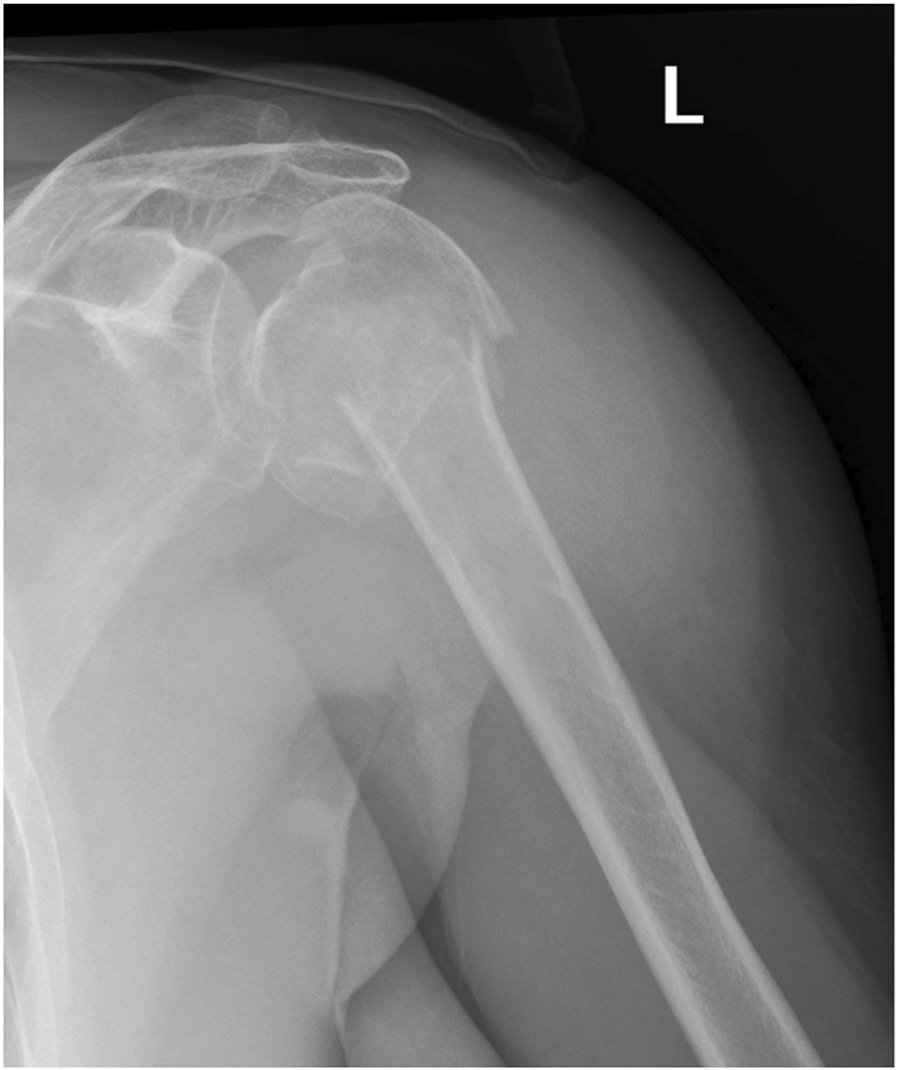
X-ray after trauma (a.-p.-view).
